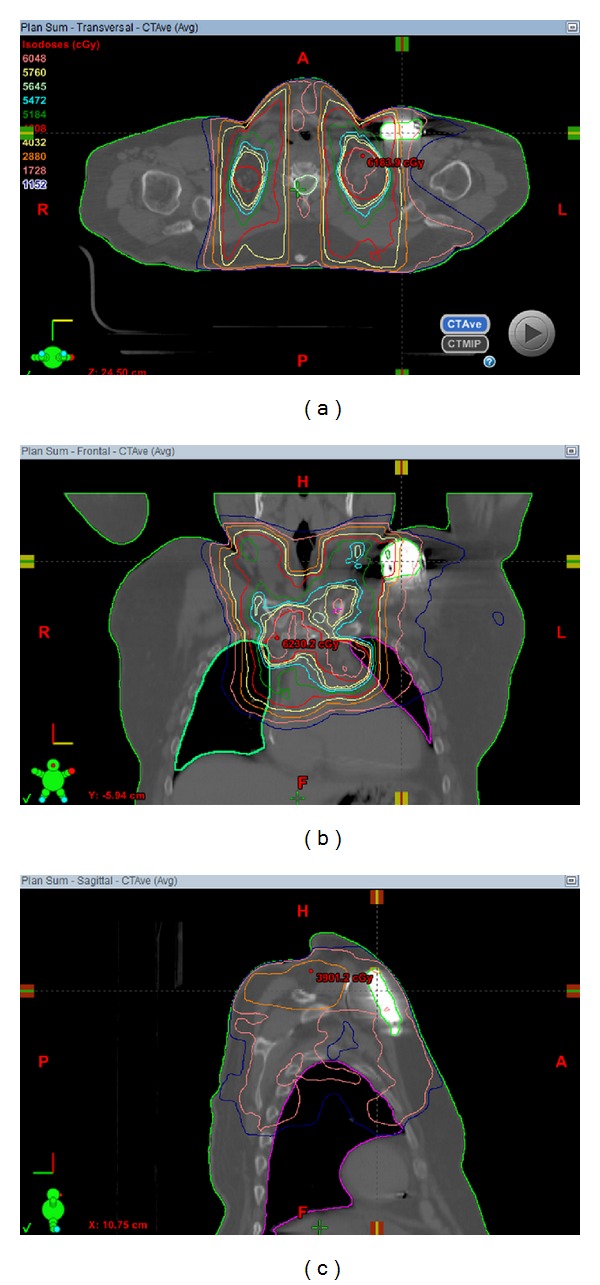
Axial (a), frontal (b), and sagittal (c) CT scans with overlying treatment volumes and corresponding doses outlined in color. AICD shown to be in field of RT.
